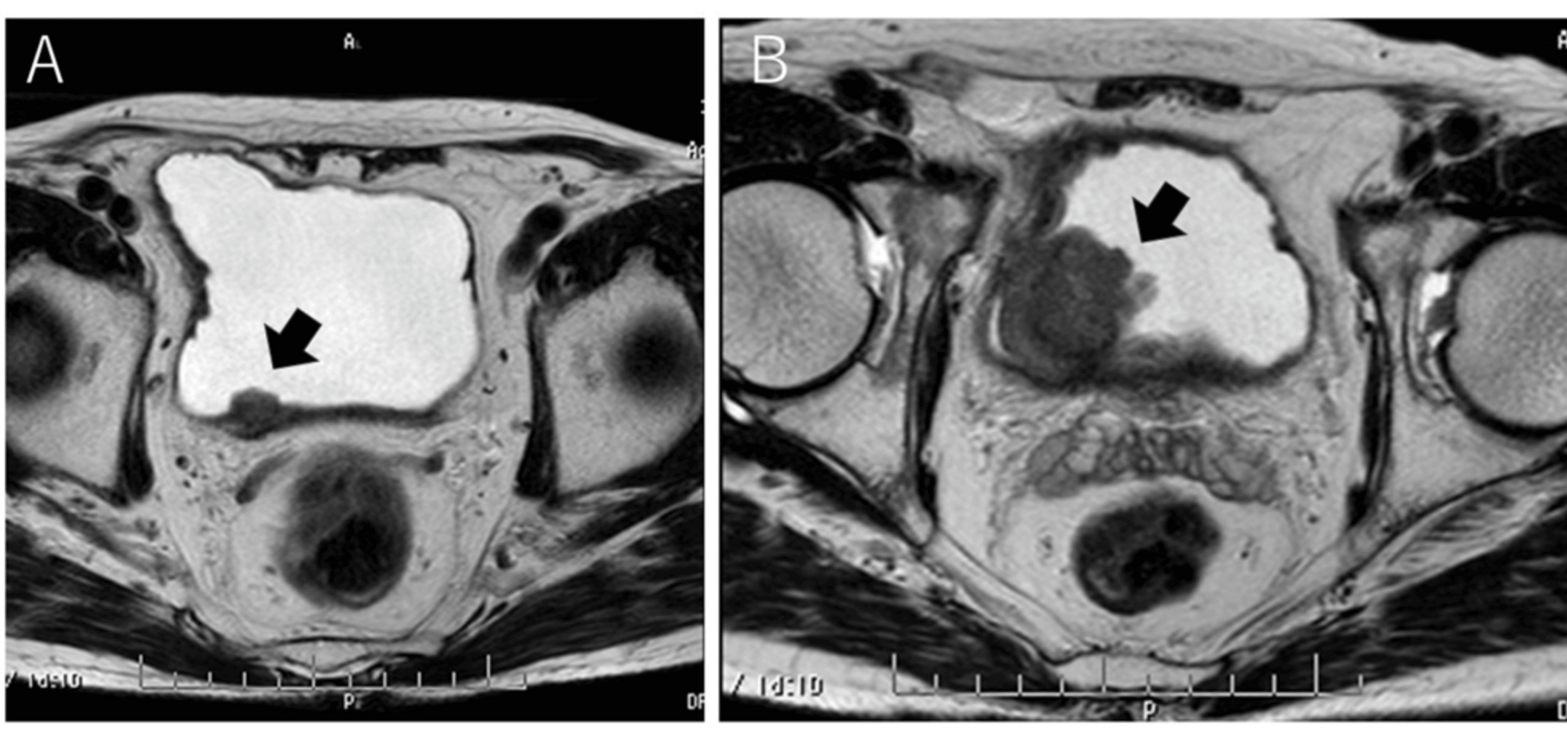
Magnetic resonance imaging findings (A) The bladder tumor (arrow) with a diameter of 15 mm at the initial visit. (B) Three months after his initial visit, the bladder tumor (arrow) has a diameter of 38 mm.
